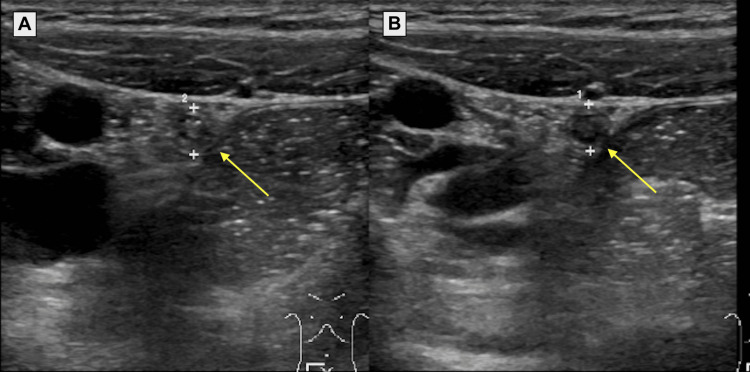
Ultrasonography of the appendix from the first (A) and second (B) emergency department visits. Panel A shows a questionably dilated appendix measuring 6.5 mm in diameter. Panel B shows a normal appendix measuring 6 mm in diameter with trace surrounding fluid. It is generally accepted that a normal appendix does not exceed 6 mm in diameter [4].
